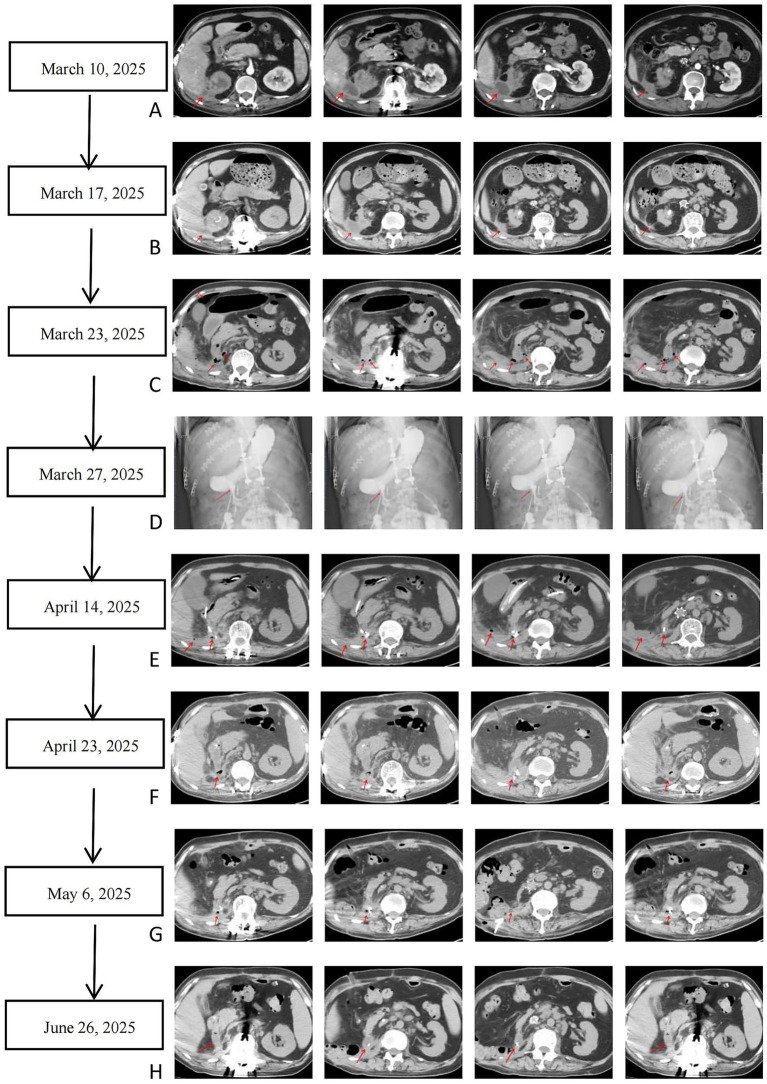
Dynamic changes of abdominal and pelvic CT images. Subfigures (A–H) show sequential CT findings (comparisons to prior scans): (A,B) Pre-nephrectomy: stable right renal calculi, double “J” stent, improved perirenal exudation (March vs. January). (C) Post-nephrectomy: right kidney absent, retroperitoneal drainage tube, left mild hydronephrosis. (D) Duodenal fistula: contrast extravasation from duodenum to abdominal cavity. (E–H) Post-fistula treatment: absorbed intra-abdominal gas, reduced sinus tract/encapsulated effusion, removed lumbodorsal catheter, improved surgical area fluid (March–May).
